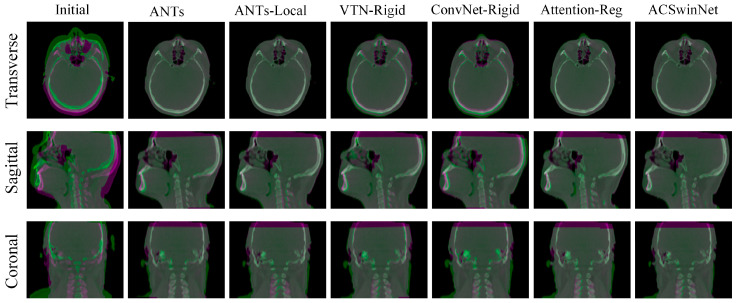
A visual comparison of image registration outcomes in a typical case of significant initial misalignment is presented. CT and CBCT images are color-coded in magenta and green, respectively, to aid visual discrimination, while the overlapped region appears gray. The results are displayed in transverse, sagittal, and coronal views. The first column represents the fusion image before registration, while the subsequent columns depict the fused images of outcomes produced by different techniques.
